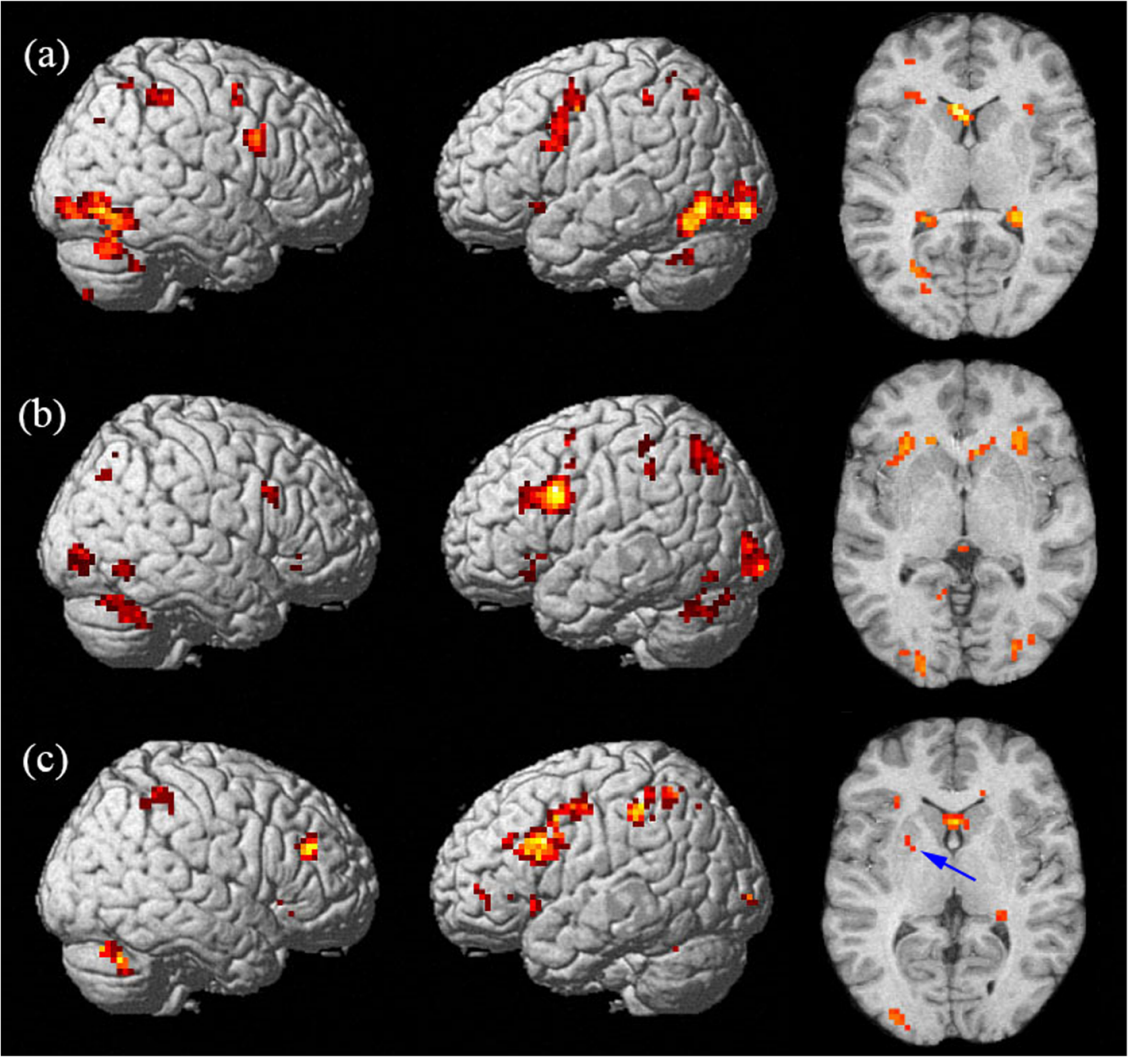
Brain activation maps superimposed onto stereotactically normalized T1-weighted image during three kinds of memory tests (*p* < 0.001, extent threshold *k* = 5). a Brain regions activated in short-memory test (STM). b Brain regions activated in long-term memory (LTM). c Brain regions activated in working memory (WM), with MrD of striatum pointed by blue arrow
